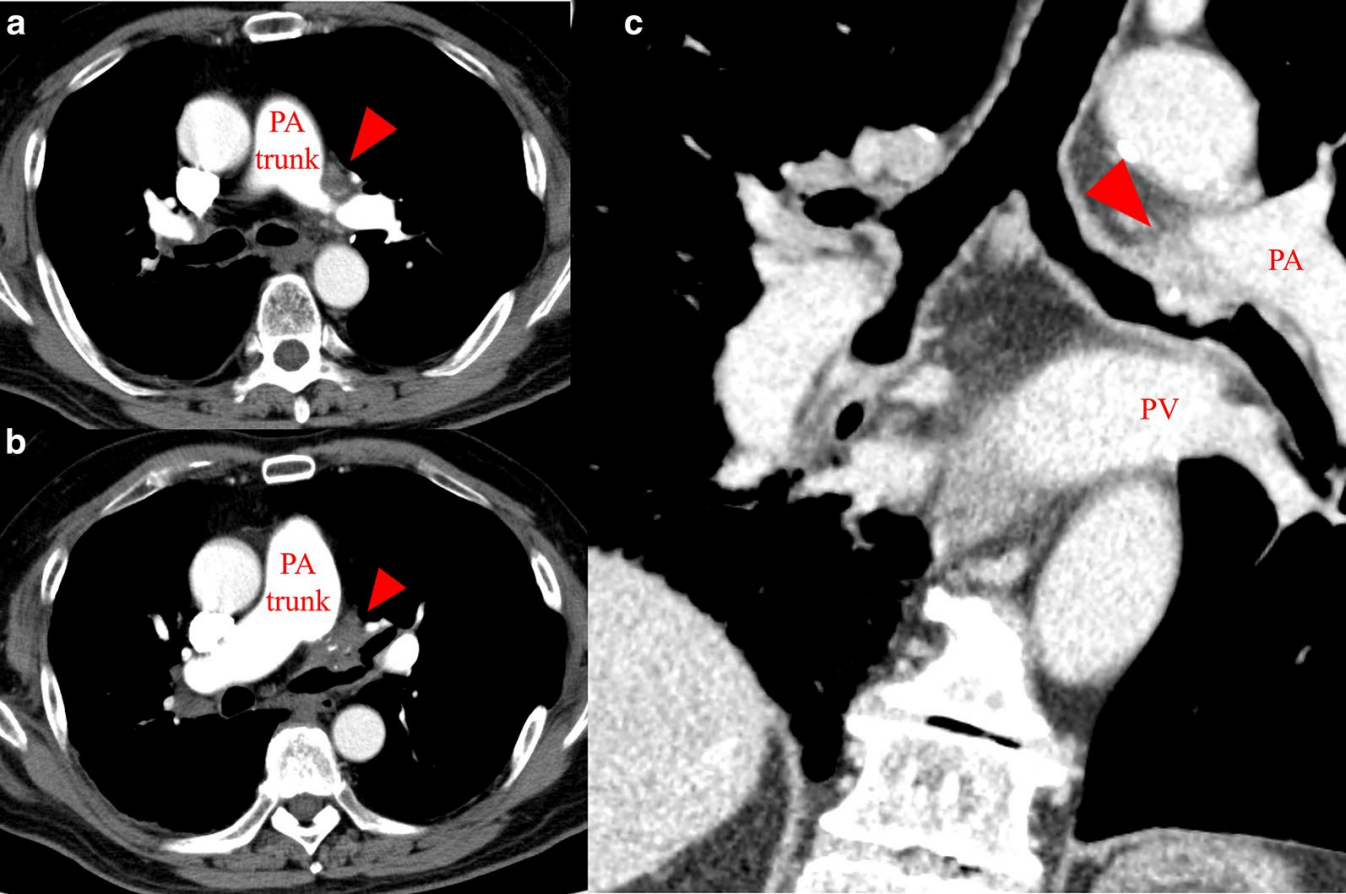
Contrast-enhanced computed tomography images. a Main PA stenosis surrounded by the foreign body in the mediastinum (red arrowhead). b The foreign body protruding to the left main bronchus (red arrowhead). c A coronal section showing the foreign body between the PA and left main bronchus (red arrowhead). *PA* pulmonary artery, *PV* pulmonary vein
